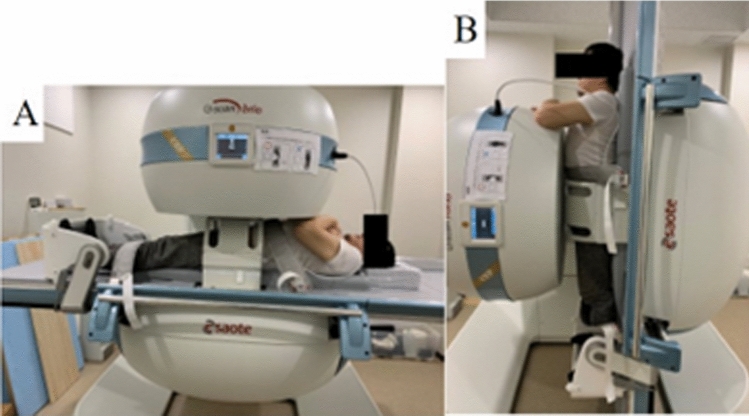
MRI was performed with the participant in the supine position A and the standing position B
